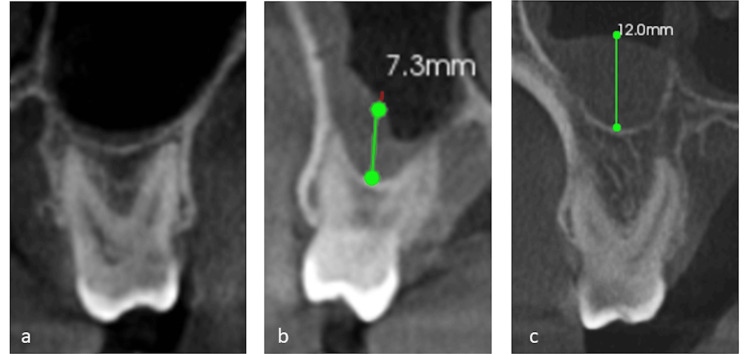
Coronal sections of cone-beam computed tomography images showing mucosal thickening grades a) Grade 1: 0-2 mm; b) Grade 2: 2-10 mm; and c) Grade 3: more than 10 mm.
